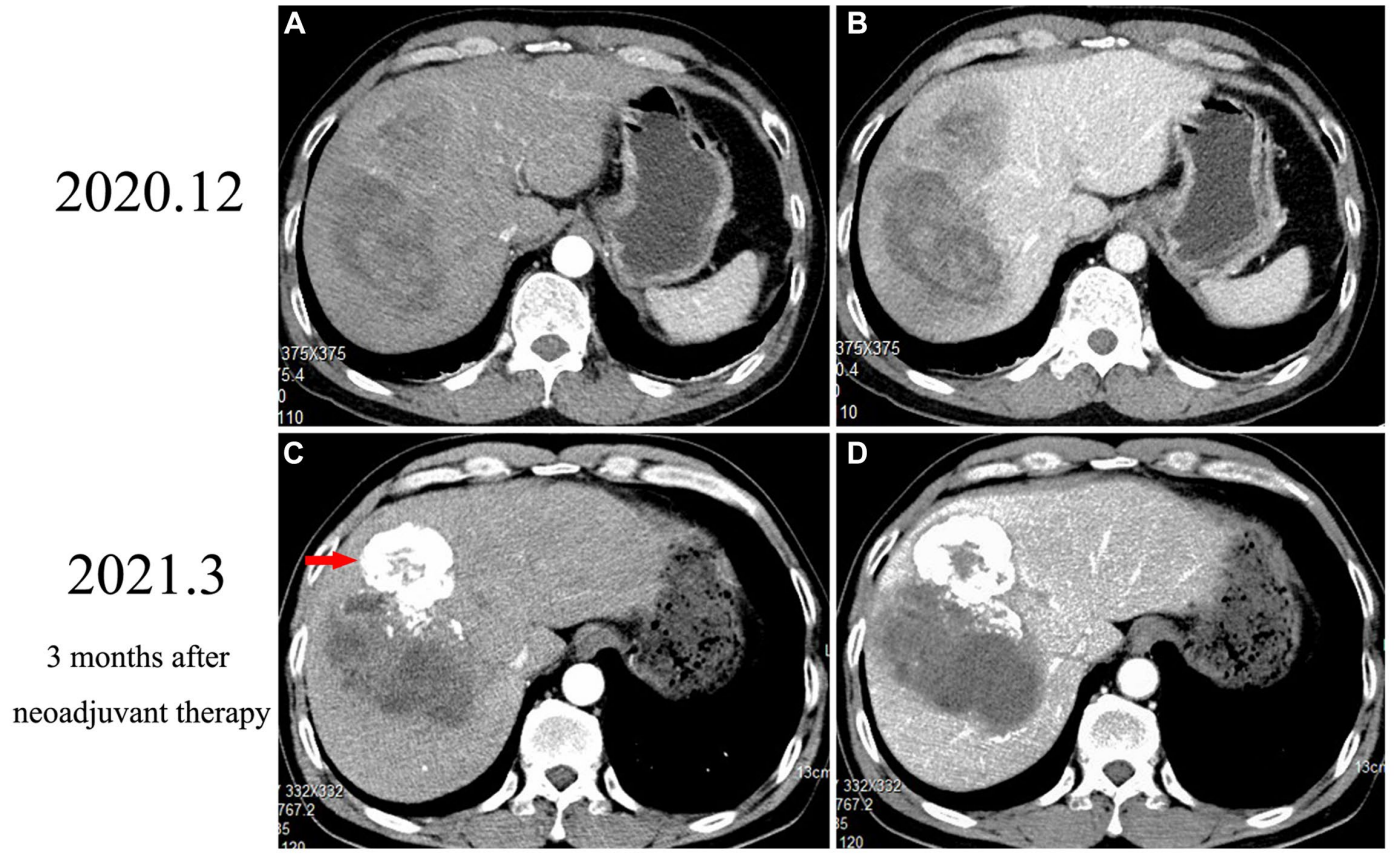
An abdominal computed tomography scan confirmed an extremely large mixed-density mass measuring approximately 13 * 10.1 * 9.2 cm located in the right liver with intratumoral hemorrhage. The tumor exhibited an enhancement pattern as fast-in and fast-out (A,B). After 3 months of neoadjuvant therapy, most of the mass was necrotic, with iodine oil deposition (red arrow) and slight local enhancement (C,D).
